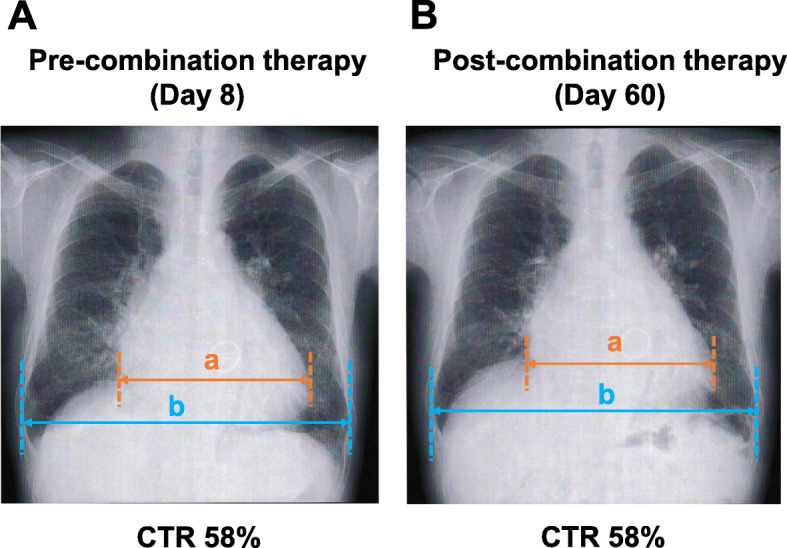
Chest X-ray of the patient pre- and post-combination therapy with midodrine and droxidopa. Cardiothoracic ratio (CTR, %) was calculated as (a/b) × 100. A Chest X-ray showing pre-combination therapy status on the 8th day of hospitalization with a CTR of 58%. B Chest X-ray showing post-combination therapy status on the 60th day of hospitalization with a CTR of 58%
